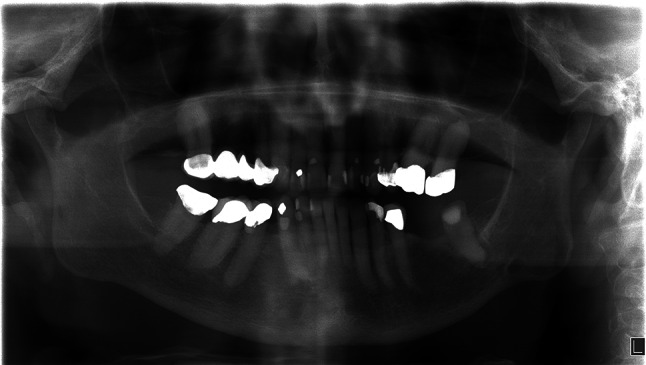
OPT radiograph showing radiolucency in the right mandible, in the region of the LL1 to LR3
